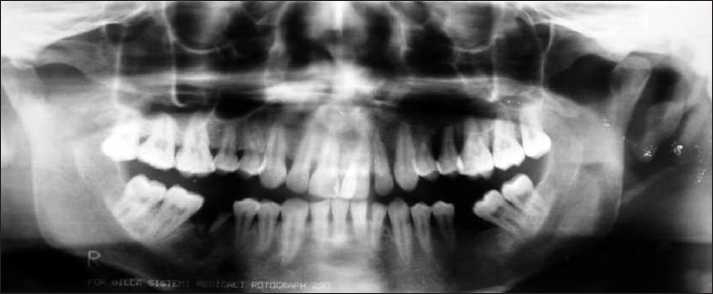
Panoramic radiograph showing a diffuse radiolucency in the maxillary left alveolar bone suggestive of loss of bone
